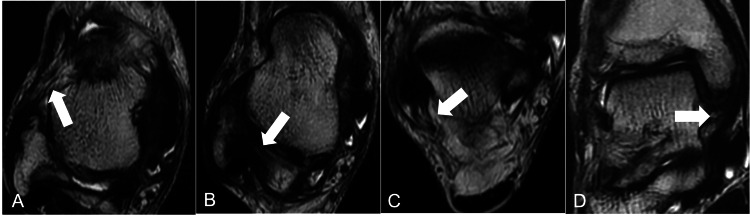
T2-weighted MRI of the patient's ankle at 11 weeks postsurgery. Axial T2-weighted MRI of the right ankle indicated scarring at the ATFL tear (A) (arrow). The PTFL (B) and CFL (C) were spontaneously repaired. The running of the DL (D) was clarified, and the tension was improved (arrow).
